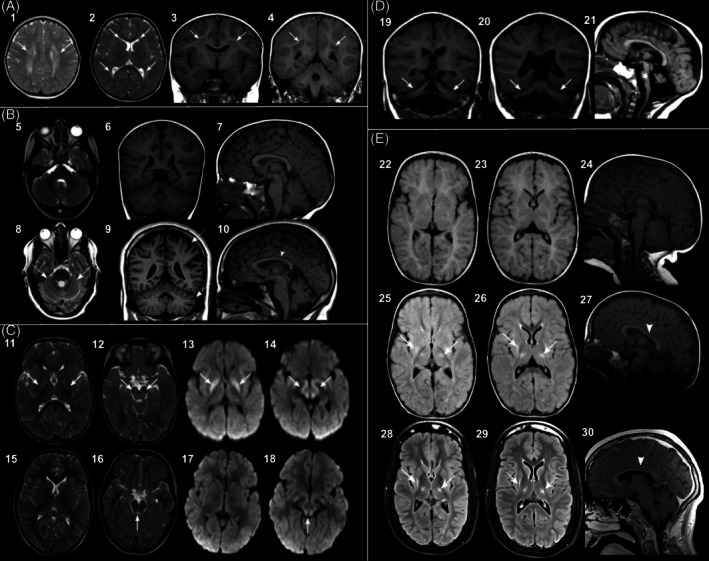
Examples of neuroradiological findings in the PEDIATAX cohort. (A) Microduplication 15q13.3 (1 year 7 months): Symmetrical periventricular and deep white matter signal changes (arrows) in axial T2 (high signal in 1, 2) and coronal T1 flair (low signal in 3, 4) images. (B) *SPAST* gene: At 3 years and 9 months (5–7) MRI was considered normal. At 18 years, MRI shows progressive cerebral and cerebellar atrophy (arrows, 8, 9), slight ventricular enlargement, and thinner corpus callosum (arrowhead 10). (C) Maple syrup urine disease, *BCKDHB* gene: During infection at 1 year and 10 months globus pallidus, cerebral peduncles and dorsal brainstem showed bilateral abnormal high T2 signal (arrows, 11, 12) and diffusion restriction (arrows 13, 14) suspicious of metabolic etiology. During infection at 10 years and 3 months MRI showed only slight T2 signal increase (arrow, 16) and slight diffusion restriction (arrow, 18) around the cerebral aqueduct and the earlier findings had normalized (15–18). (D) Microdeletion Xp11.4 (9 months): Severe pontocerebellar hypoplasia with a typical dragon fly sign (arrows) in coronal images (19, 20). Both cerebellar hemispheres and vermis are hypoplastic. Marked pontine hypoplasia (star) is demonstrated in the sagittal plane (21). (E) Cerebral palsy: At 5 months brain MRI was considered normal in a child with diagnosis of cerebral palsy (22–24). At 2 years and 6 months there were signs of Wallerian degeneration in the corticospinal tracts and atrophy and increased T2 signal bilaterally in thalami and nuclei lentiformis (arrows 25, 26) and around the posterior horns of lateral ventricles. In addition, corpus callosum was thinner than normally (arrowhead 27). The findings persisted at 17 years (28–30).
